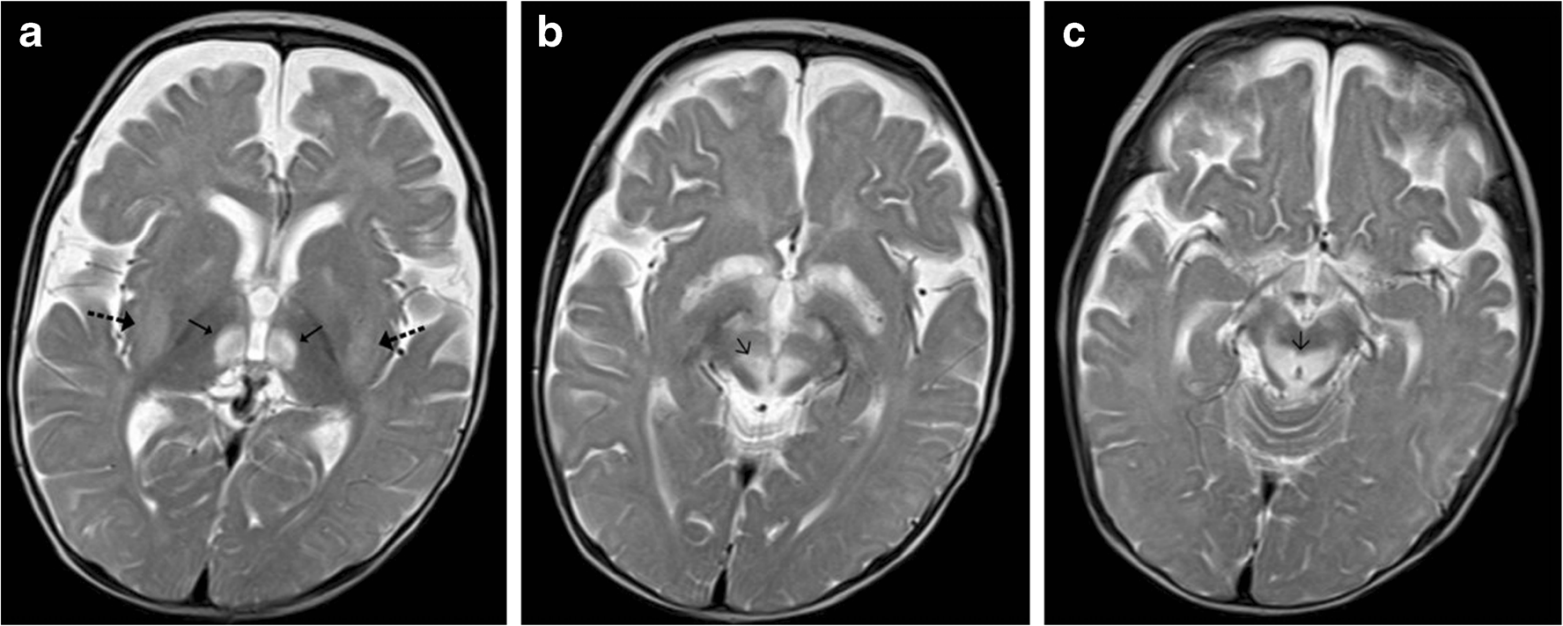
A 9-month-old child with delayed milestones and seizures: a–c Axial T2-weighted images show hyperintense signal involving the putamina (*dotted arrow*) and medial thalamic nucleus. Caudally there is involvement of the mid brain (*short arrow*) with characteristic involvement of the periaqueductal region in this patient with Leigh syndrome
